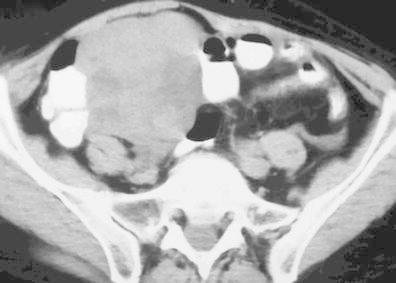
Undifferentiated ovarian carcinoma in a 59-year-old BRCA2-mutation carrier woman. CT scan demonstrates a large multilobulated complex cystic-solid right adnexal mass. There were neither ascites nor enlarged lymph nodes
